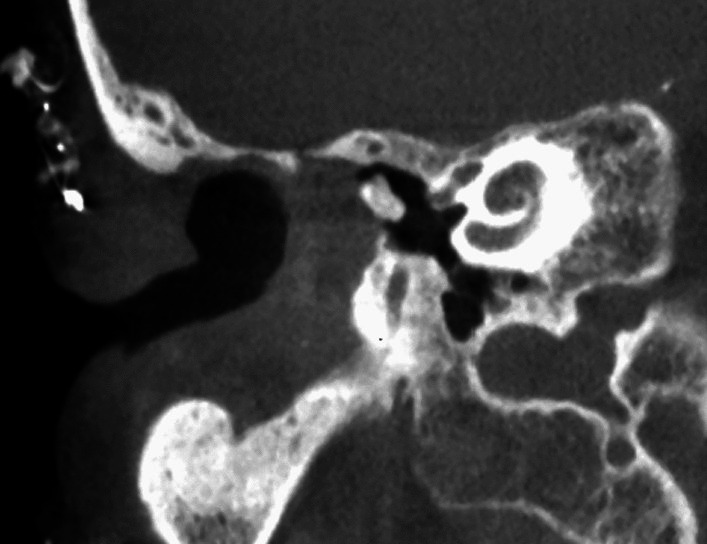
Preoperative coronal CBCT of the petrous
